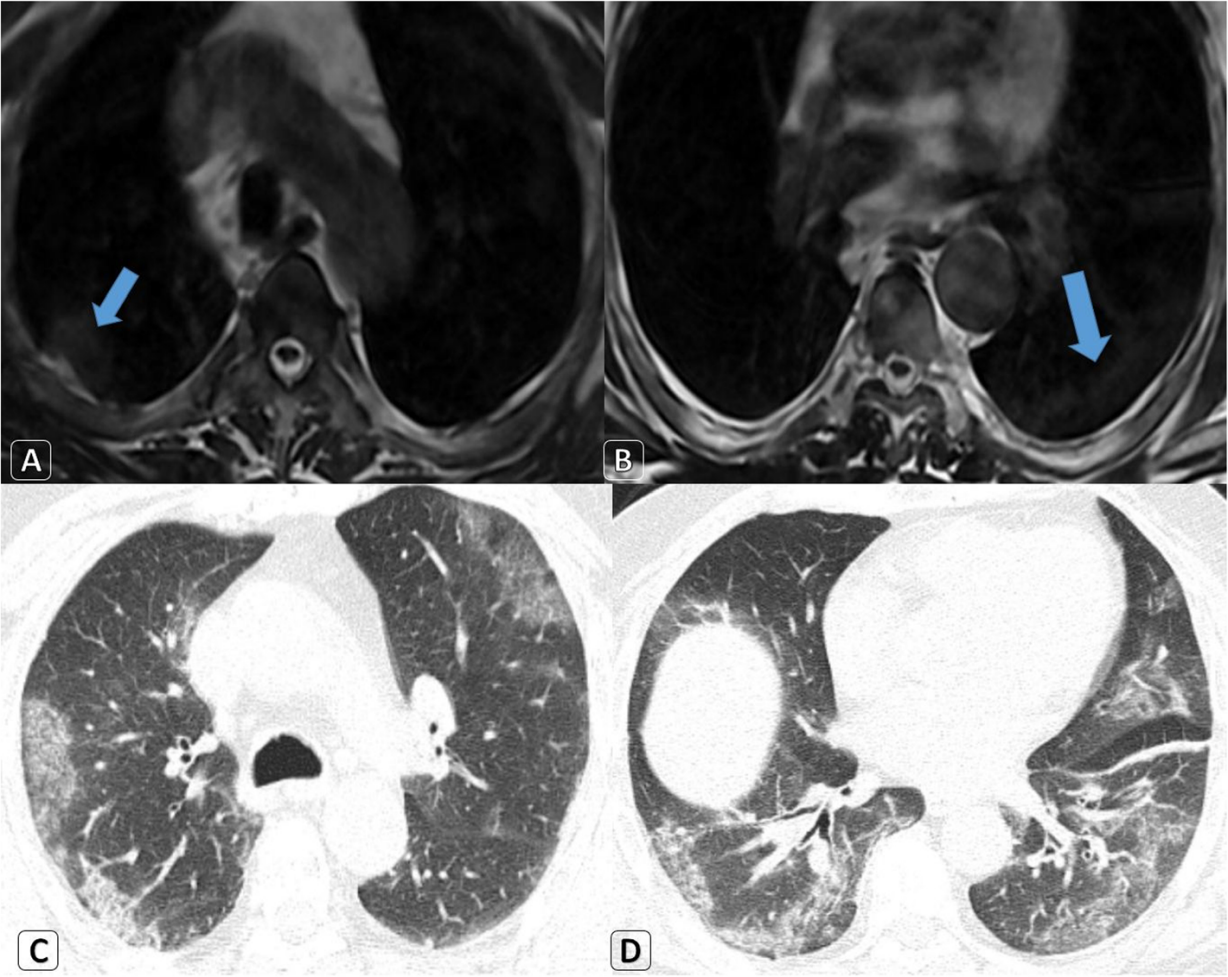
A 58-year-old male patient with history of prostatic cancer. He had a visit to the radiology unit to do a whole spine MRI because of back pain. a-b Dorsal spine MRI using high standard infection control measures revealed: axial T2WI showed the same patches with minimal overlying pleural reaction (blue arrows). c-d Screening axial chest HRCT lung window revealed bilateral large peripheral located ground-glass opacities mixed with consolidative changes. Clinical decision was isolated hospitalization, medical treatment, and PCR testing. Positive PCR result for COVID-19 was proved later
